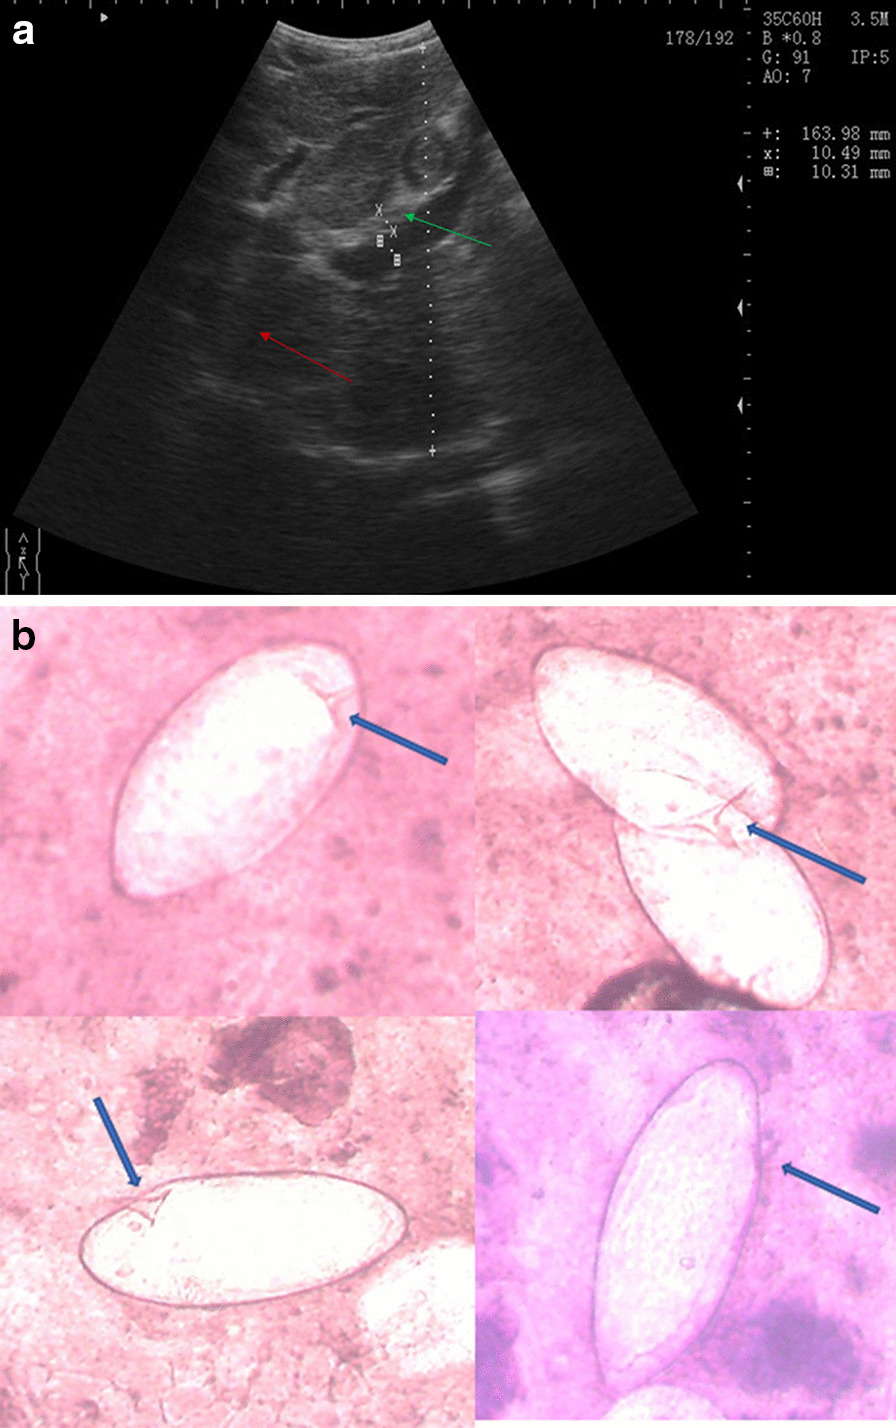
a Ultrasound examination of patient #1: fibrotic liver pattern showing decreased definition of portal vein wall (green arrow) and a heterogeneous echotexture of liver parenchyma (red arrow). b
